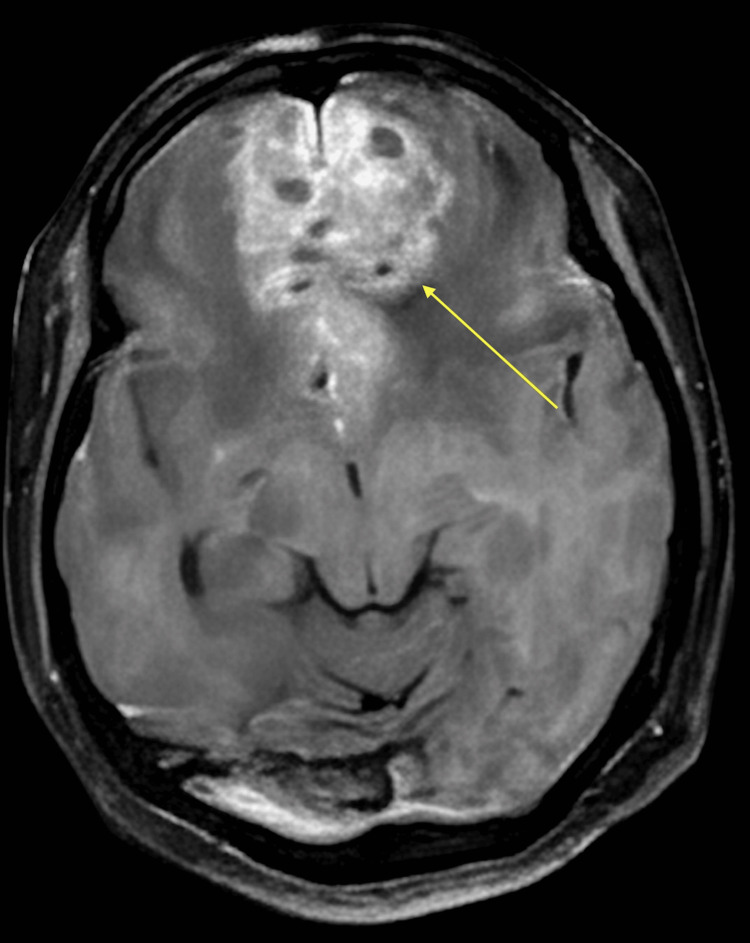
Contrast-enhanced axial MRI brain showing a heterogeneously enhancing solid-cystic mass crossing the genu of the corpus callosum with bifrontal extension and perilesional edema, suggestive of butterfly glioblastoma multiforme The yellow arrow indicates the enhancing lesion in the left frontal parasagittal region, with adjacent parenchymal compression and local mass effect.
